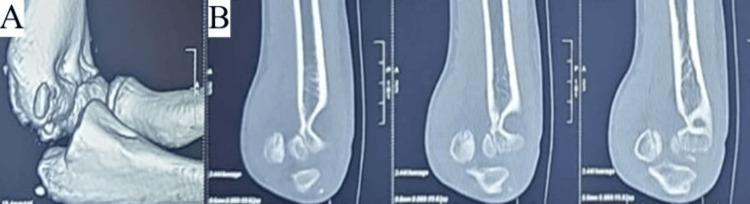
(A) Post-reduction CT of the elbow joint, 3D reconstruction view. (B) Sagittal view CT of the elbow joint CT, computed tomography
